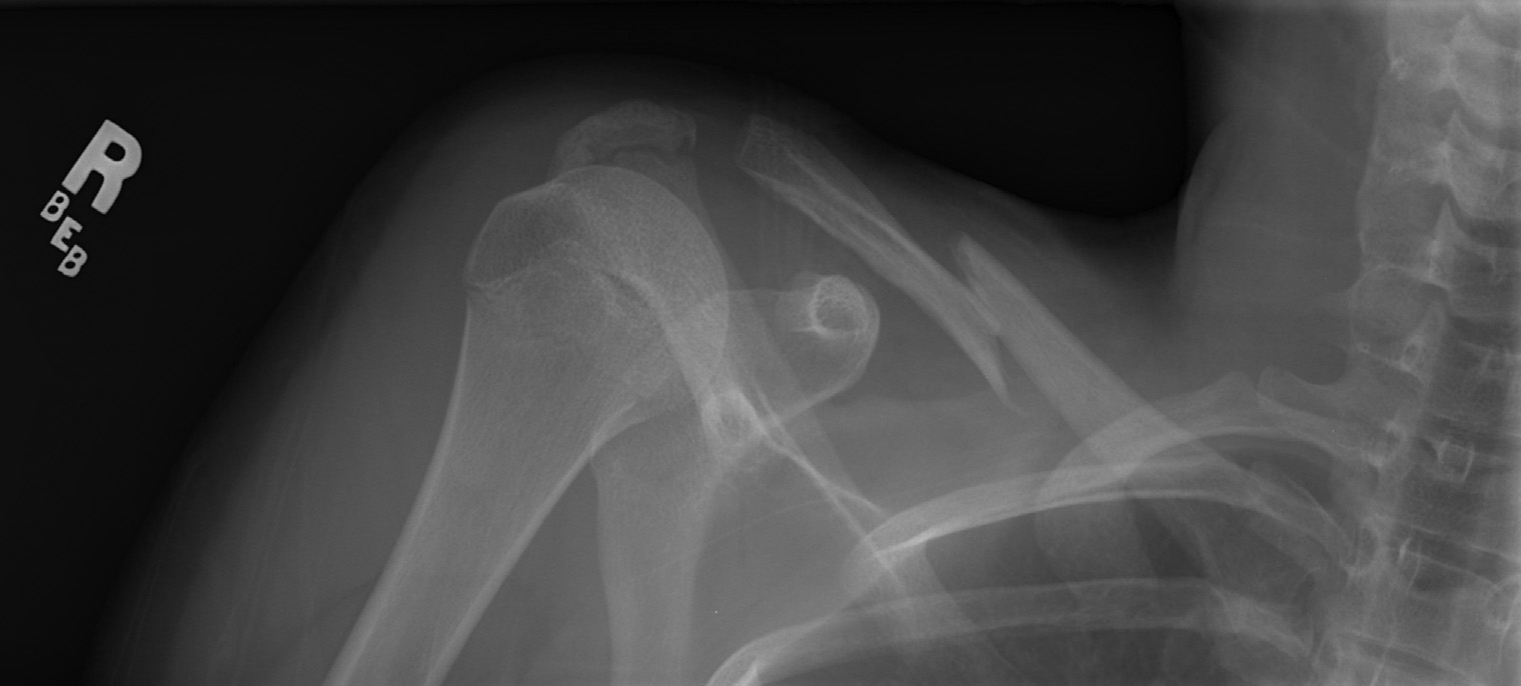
Anterior-posterior uptilt radiograph of original right displaced midshaft clavicle fracture of patient at 13 years old.
